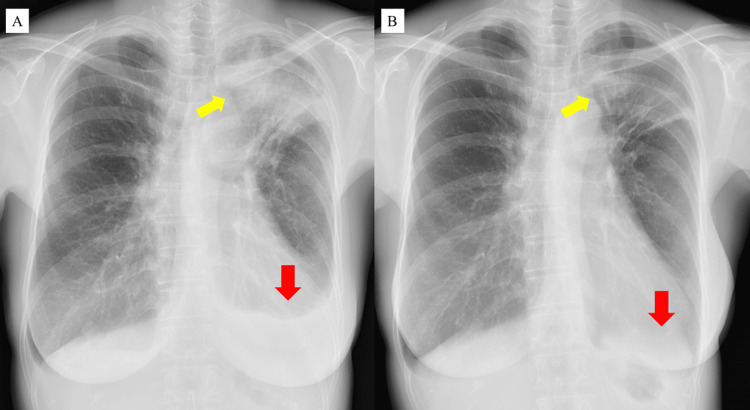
Chest radiography before and after the first treatment Chest radiography shows infiltration shadows in the left upper lung and left pleural effusion (yellow and red arrow) (A). Following the administration of oral prednisolone (10 mg/day) and levofloxacin hydrate (500 mg/day) (after five days), the infiltration shadows improved, and the left pleural effusion had disappeared (B)
